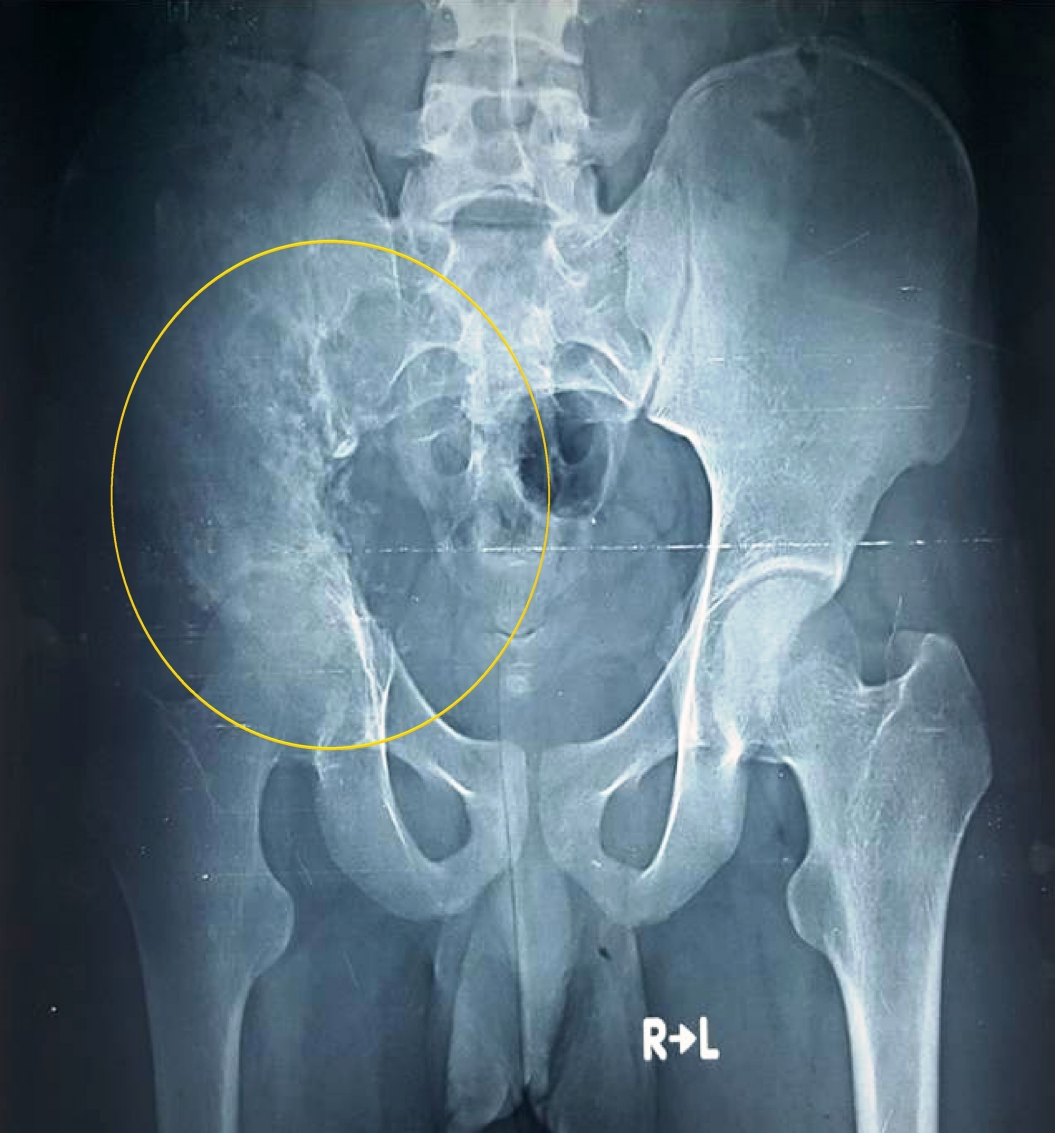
Pelvic radiograph showing osteolytic lesions in the right ilium, ischium, and sacroiliac and hip joints (anteroposterior view; circle)
